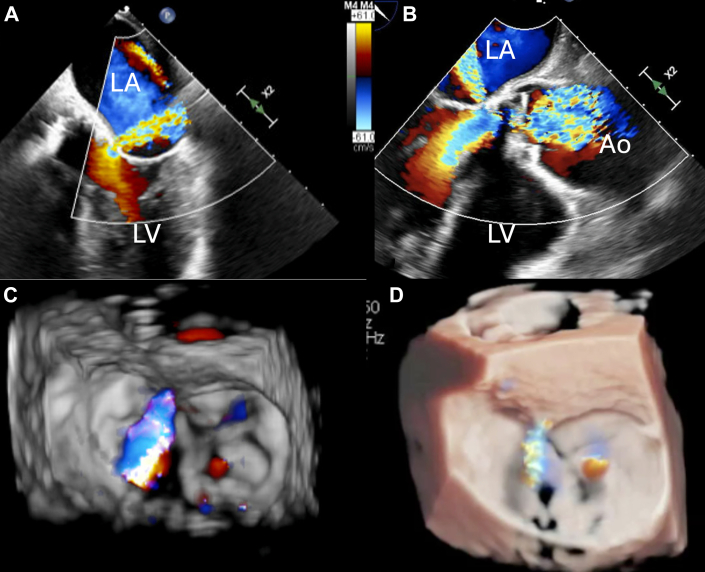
Transesophageal Echocardiogram (A and B) LVOT-LA fistula via the zone of mitral-aortic intervalvular fibrosa (fibrous aortomitral curtain). (C and D) Three-dimensional rendering of the LVOT-LA fistula. Ao = aorta; LA = left atrium; LV = left ventricle; LVOT = left ventricular outflow tract.
